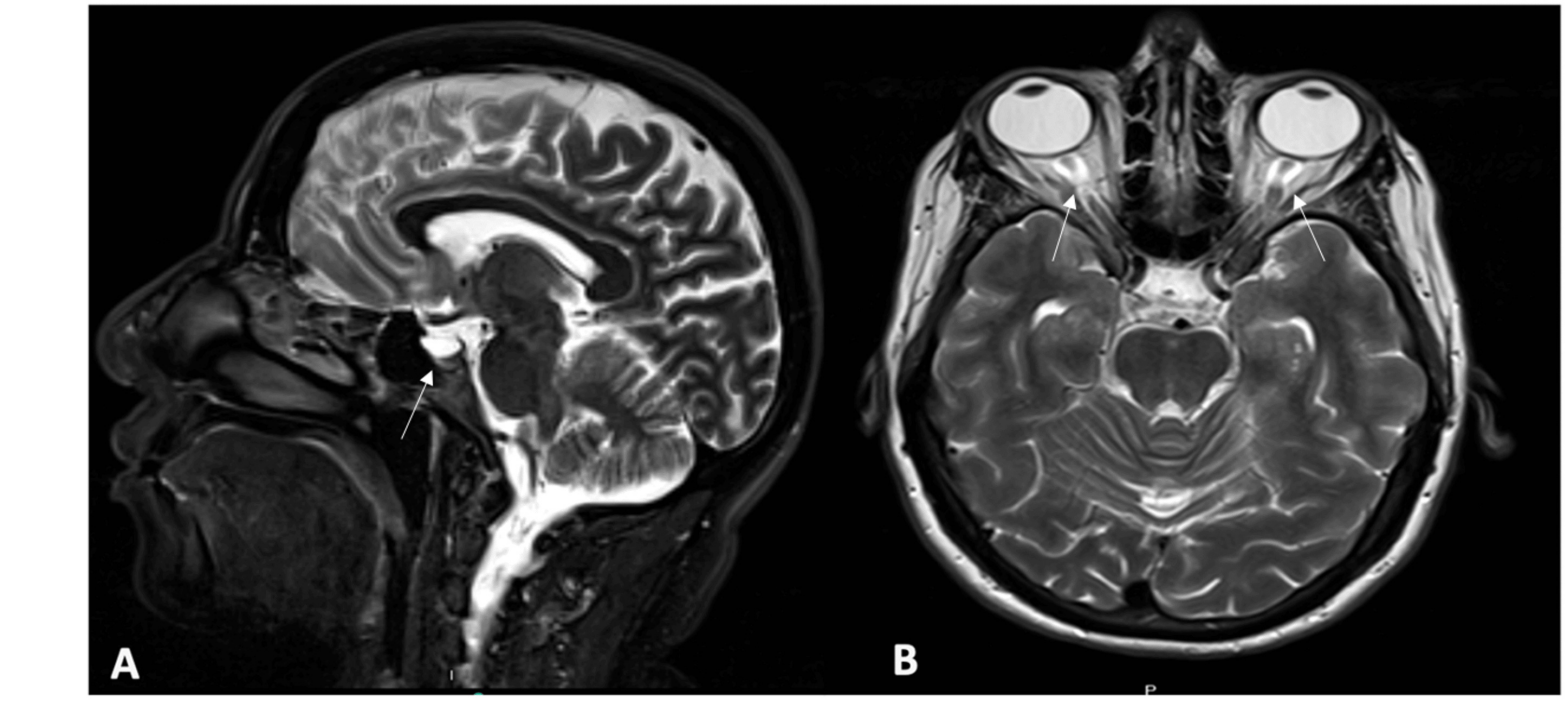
Brain magnetic resonance imaging. Brain MRI T2 fluid-attenuated inversion recovery sequence sagittal (A) and axial (B) views showed partially empty sella turcica and prominent subarachnoid space around the optic nerve.
